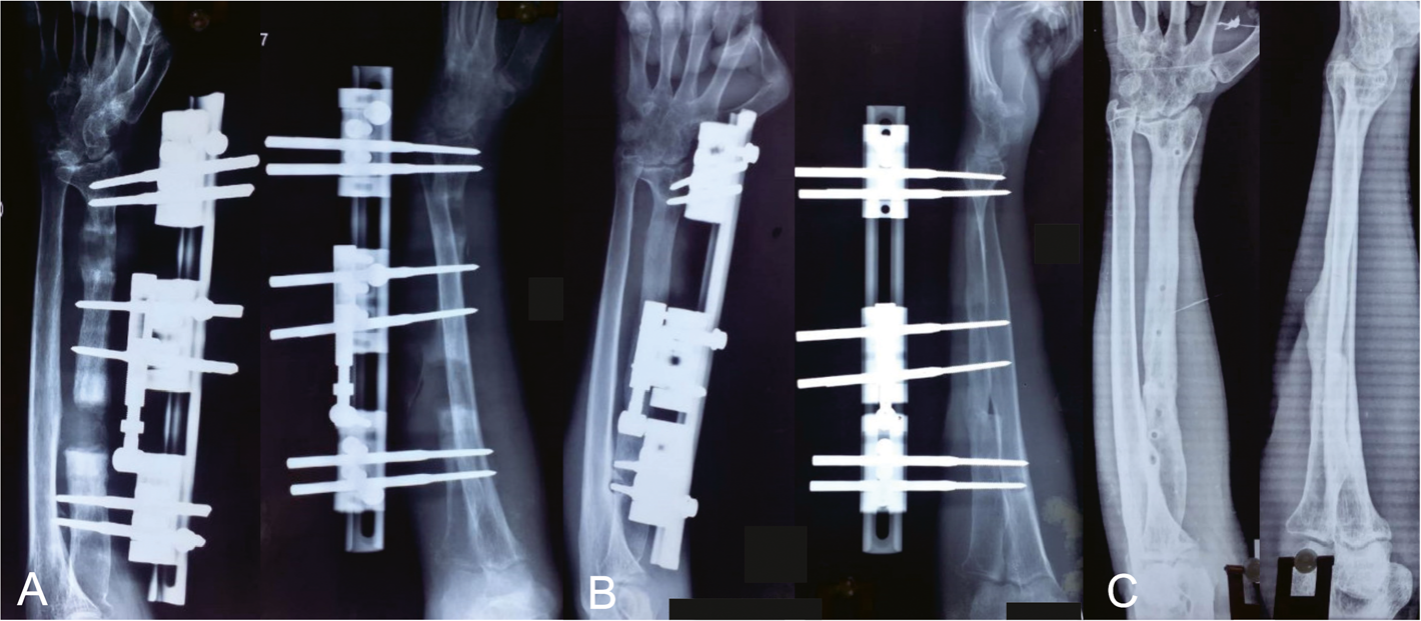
X-rays of the bone transport procedure. a One month after the operation with bone transport. b Eight months after the operation with bone transport, consolidation of the regenerate and union at the docking site was shown. c AP and lateral views of X-rays after removing the external fixator
